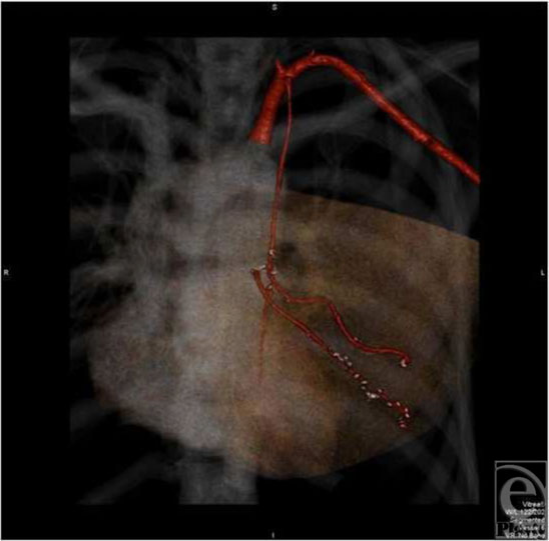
Computed tomographic angiogram performed on postoperative day 5 showing that both antegrade and retrograde arteries are patent.
